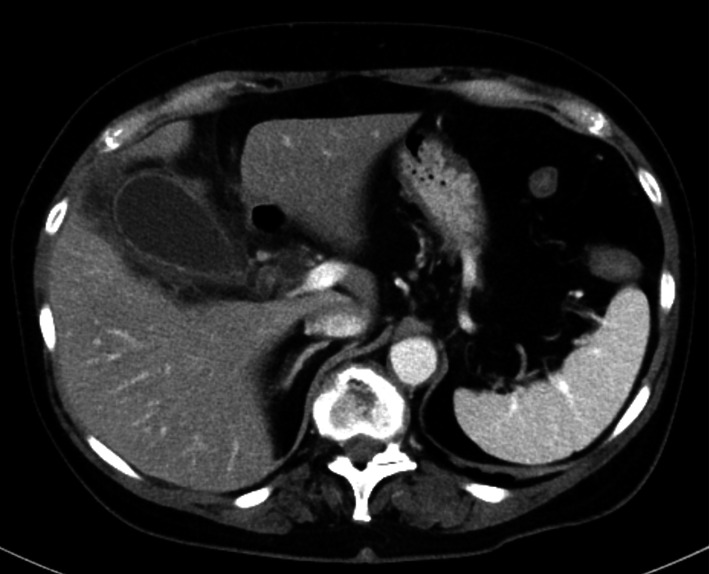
Contrast-enhanced computed tomography. Image shows gallbladder distention, wall thickening, and pericholecystic inflammation
